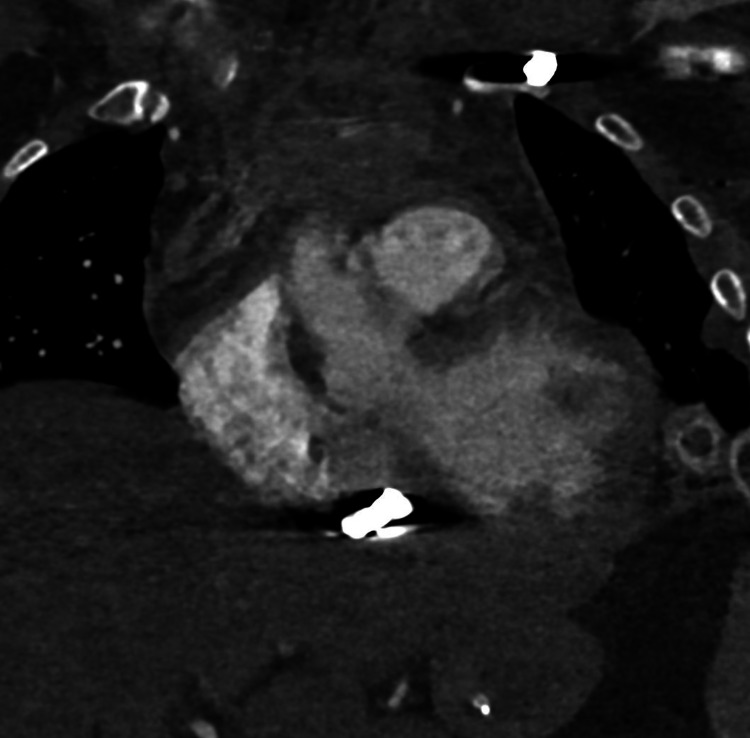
Anteroposterior CT of the chest. Displays two hyperattenuated signals: one localizing the bullet in the middle mediastinum inferior to the heart and the other located in the left shoulder, identifying the bullet that lacerated branches of the left subclavian vein. CT: computed tomography
